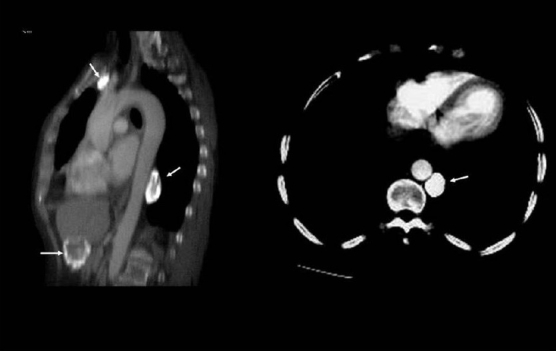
Hydatid cysts do not normally calcify within the lungs; two calcified hydatid cysts in the superior and posterior mediastinum are seen. Note the calcified hydatid cyst within the left lobe of the liver on the coronal CT reconstruction
